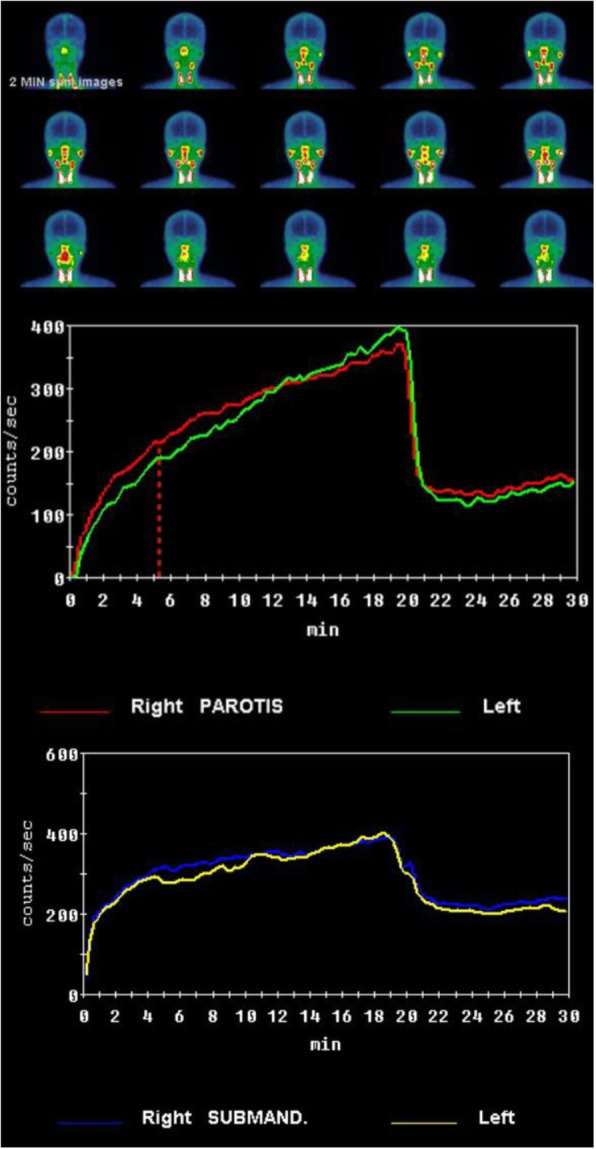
Salivary gland scintigraphy
